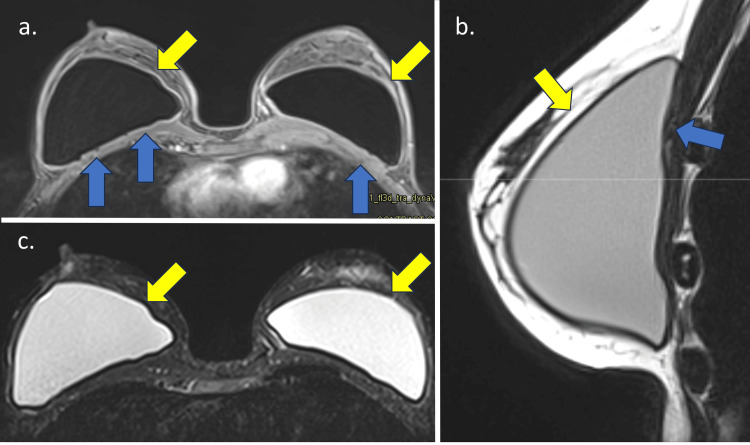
Breast MRI sequences showing MRI fibrous capsule Baker classification Grade 2 in a 42-year-old patient with silicone implants for four years. The Baker classification was Grade 2. (a) Axial T1 post-contrast sequence shows fibrous capsular thickening with contrast enhancement (yellow arrows) associated with black-drop signal (blue arrows); (b) The sagittal proton-density sequence shows the same findings; (c) Axial STIR sequence confirms the capsular thickening. STIR: short tau inversion recovery Image Credit: Eduardo de Faria Castro Fleury
